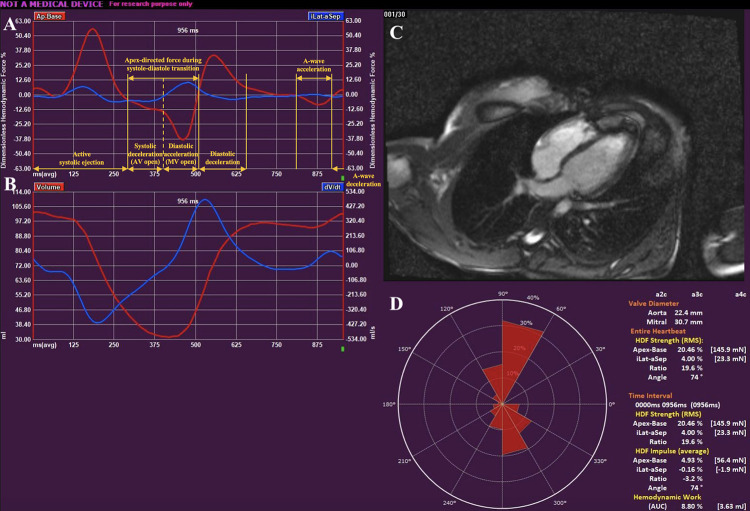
Hemodynamic forces analysis. (A) HDF curves (A-B in red; L-S in blue). Several time intervals can be defined from the HDF curve including active systolic ejection, systolic deceleration, diastolic acceleration, diastolic deceleration, atrial thrust, and atrial deceleration; (B) LV volume and rate of changes in LV volume curve; (C) Cardiac MRI image; (D) Polar histogram depicting the distribution and magnitude of LV HDFs. The magnitude (red triangles) and directional distribution (angles) of HDF across the entire heart cycle are both depicted on a polar histogram. The top of the histogram (90 degrees) is used to represent the LV base, while the bottom (270 degrees) indicates the LV apex.
